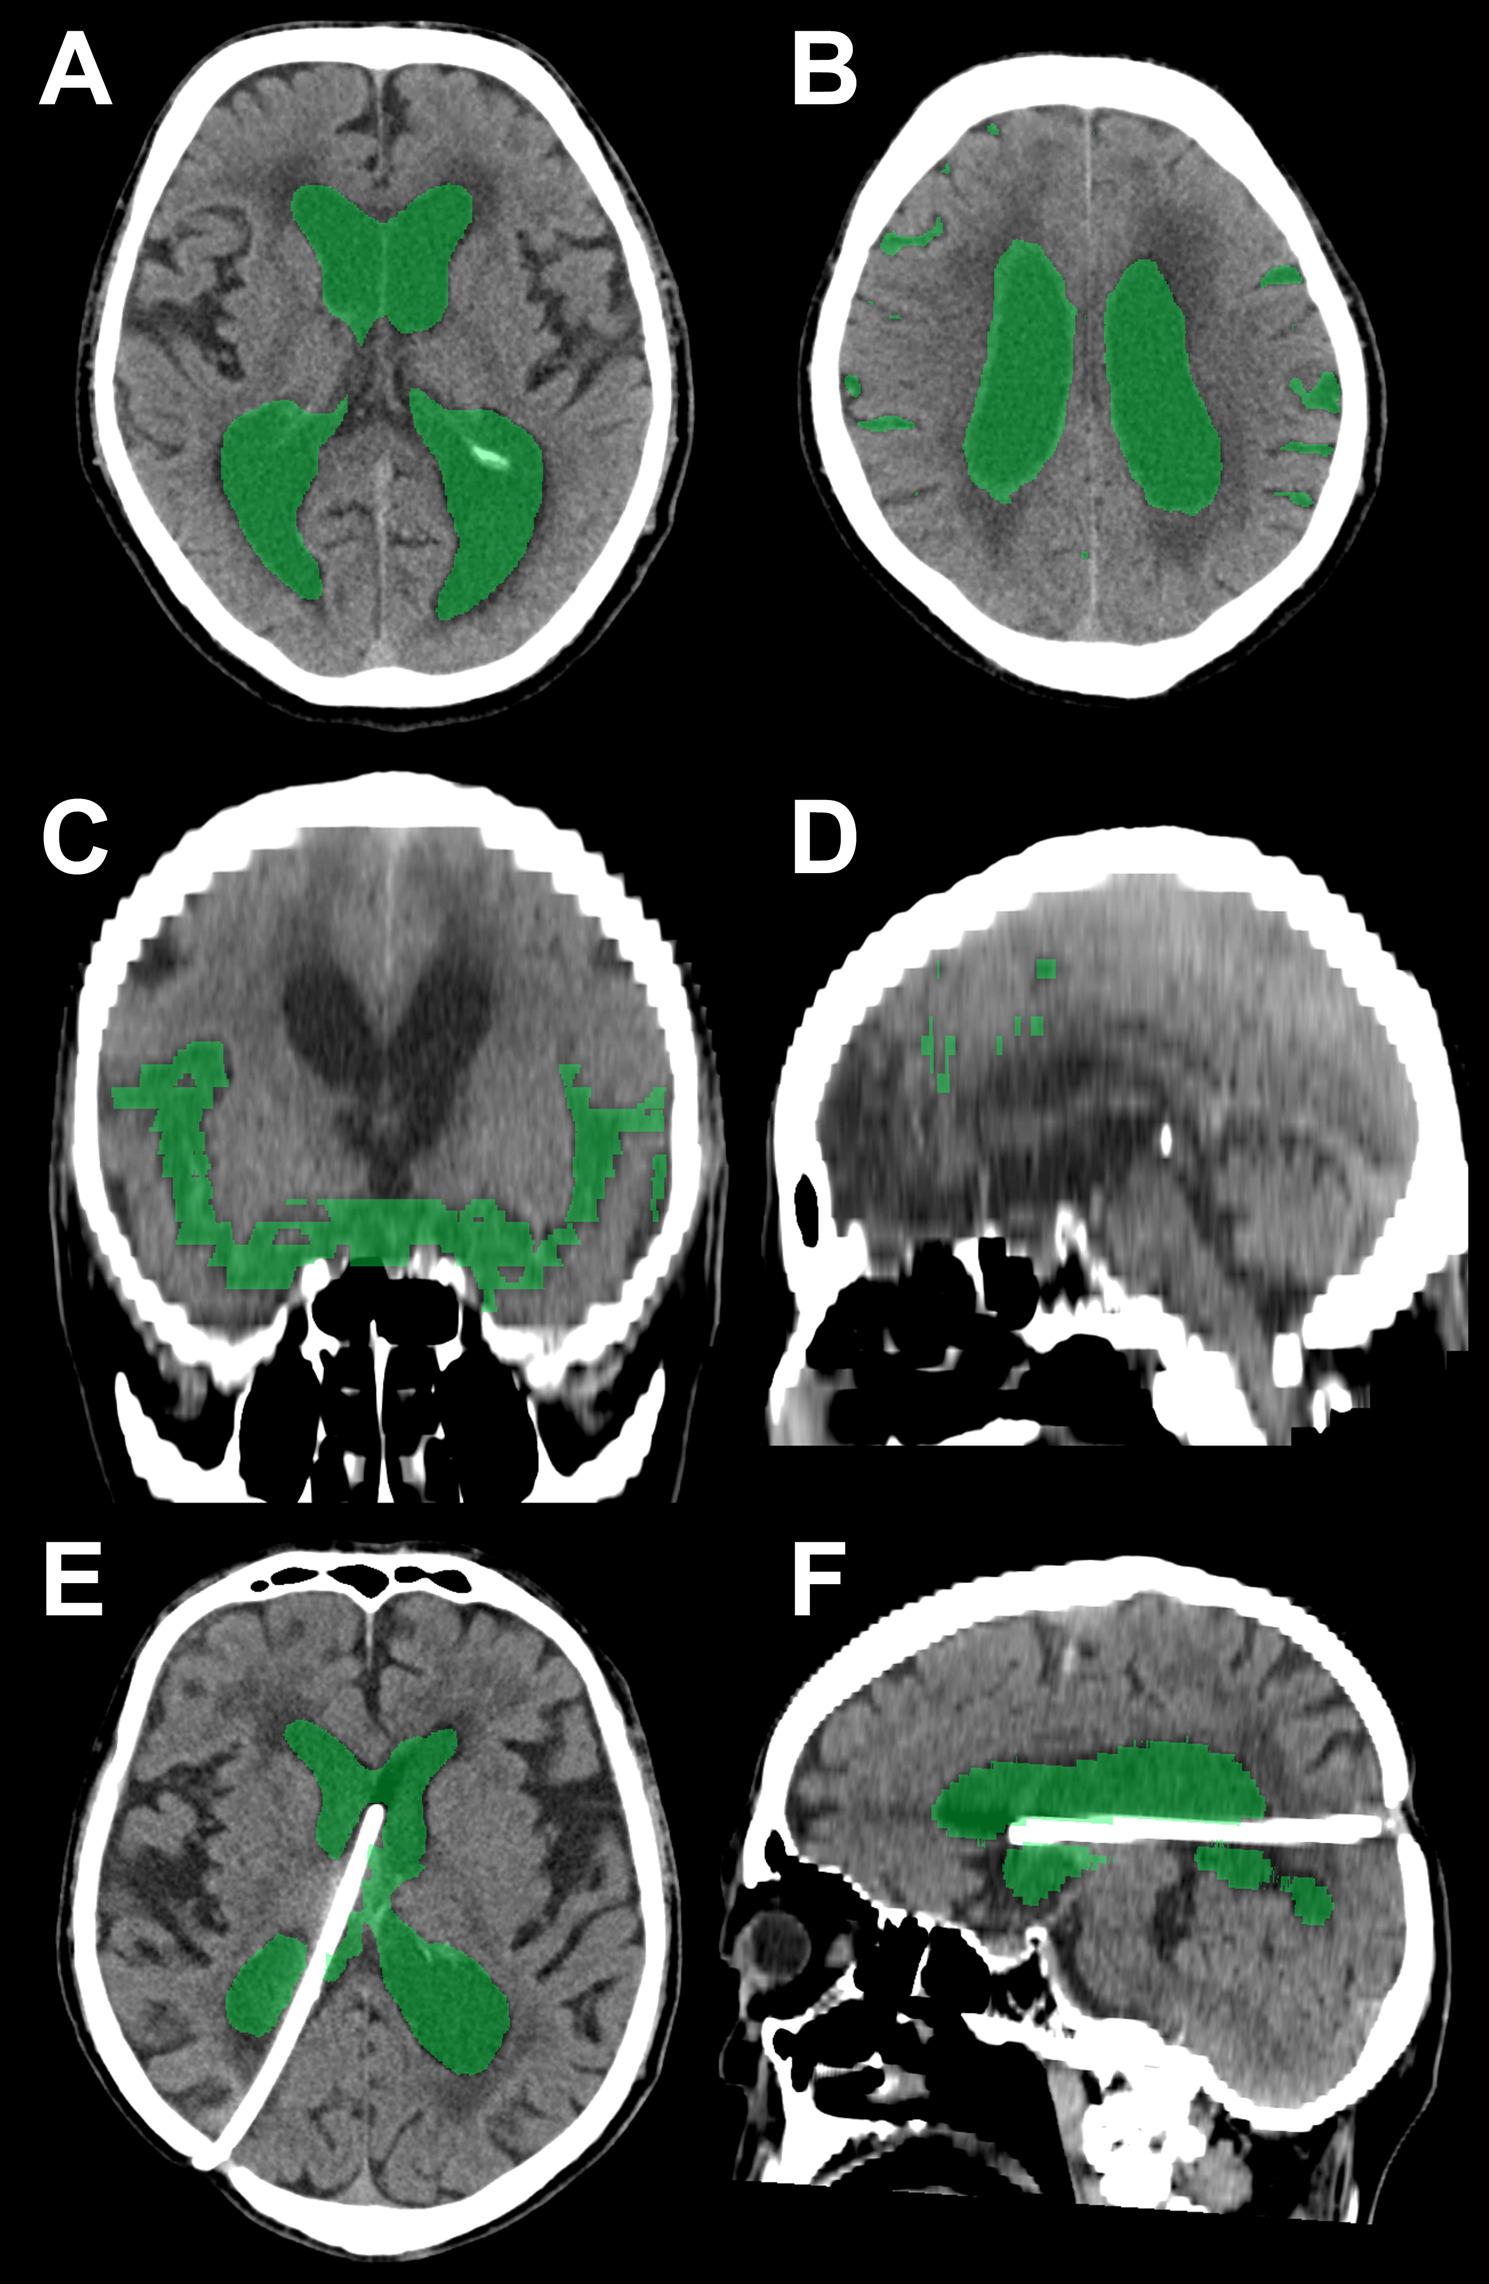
Region Extraction by Semantic Segmentation Using the Final AI Model (Version 10). A, B: Total ventricular regions (green) extracted from 5.0-mm plain CT images of a patient with iNPH. C: Sylvian fissures and basal cisterns (green) segmented from the same 5.0-mm CT scan. D: High-convexity subarachnoid space (green) segmented from the same 5.0-mm CT scan. E, F: Total ventricular region (green) extracted from a 2.0-mm postoperative CT scan of the same patient in axial (E) and sagittal (F) views.
